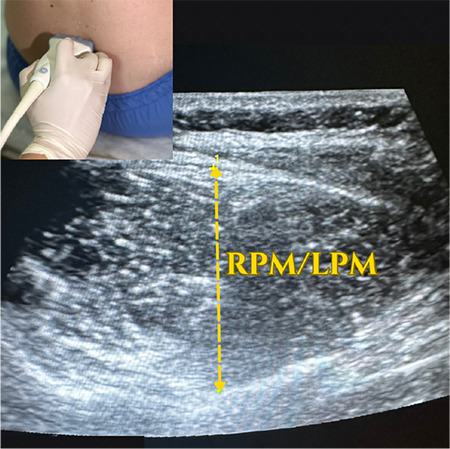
USG image of examined lumber vertebral region. USG, ultrasonography; RPM, right paraspinal muscle; LPM, left paraspinal muscle.
